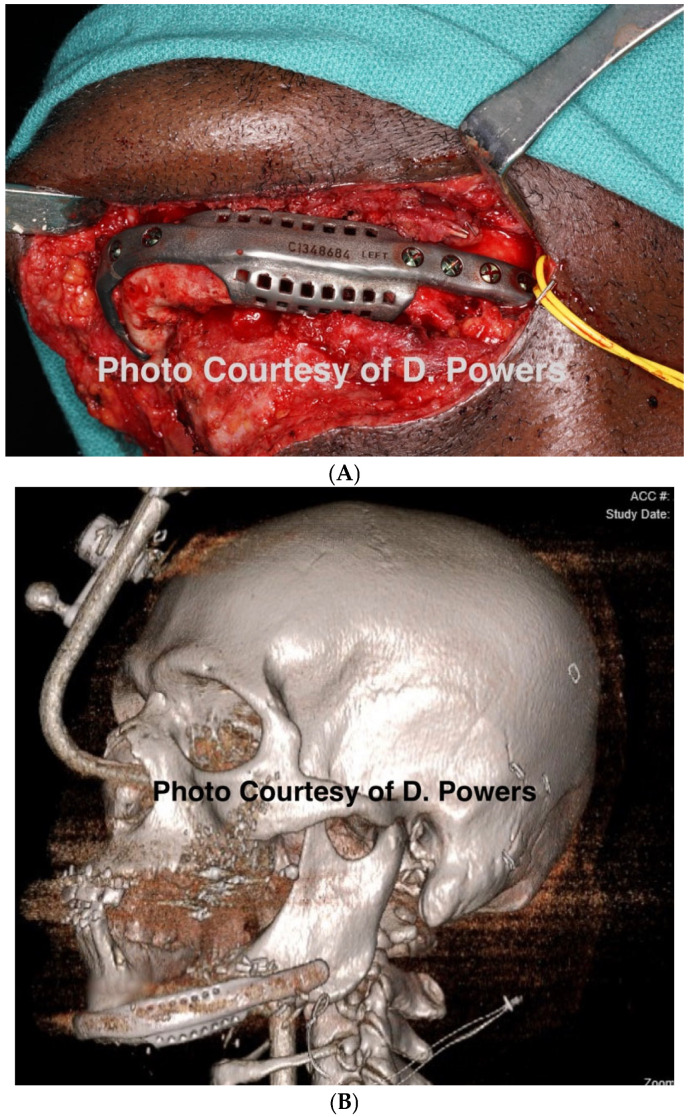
(A) Milled plate for mandibular reconstruction of gunshot wound. (B) Intraoperative computed tomography scan verifying anatomic placement.
